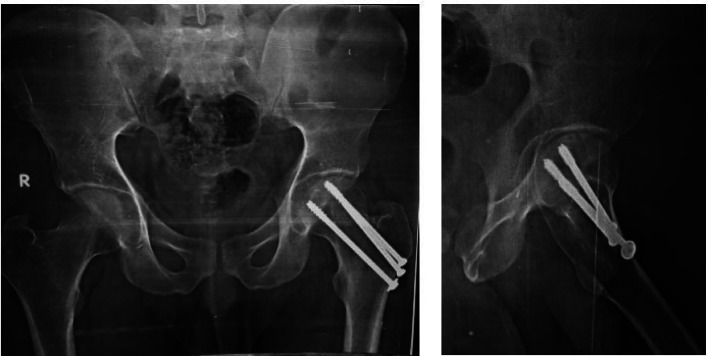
Follow up x-rays at 12th months of the patient treated by MCCS
